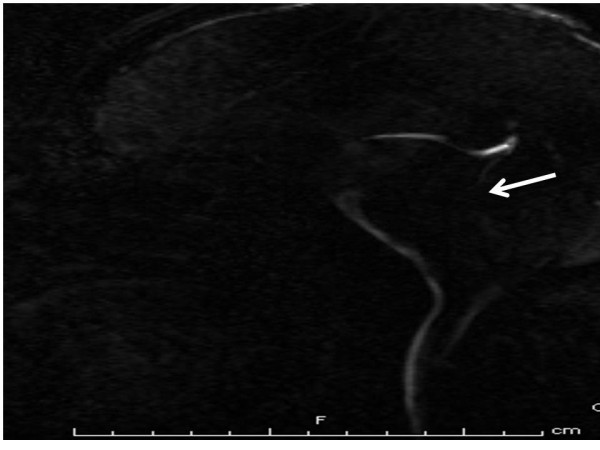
Cine flow MRI: lack of cerebral aqueduct flow void (arrow) but no obvious cyst.
